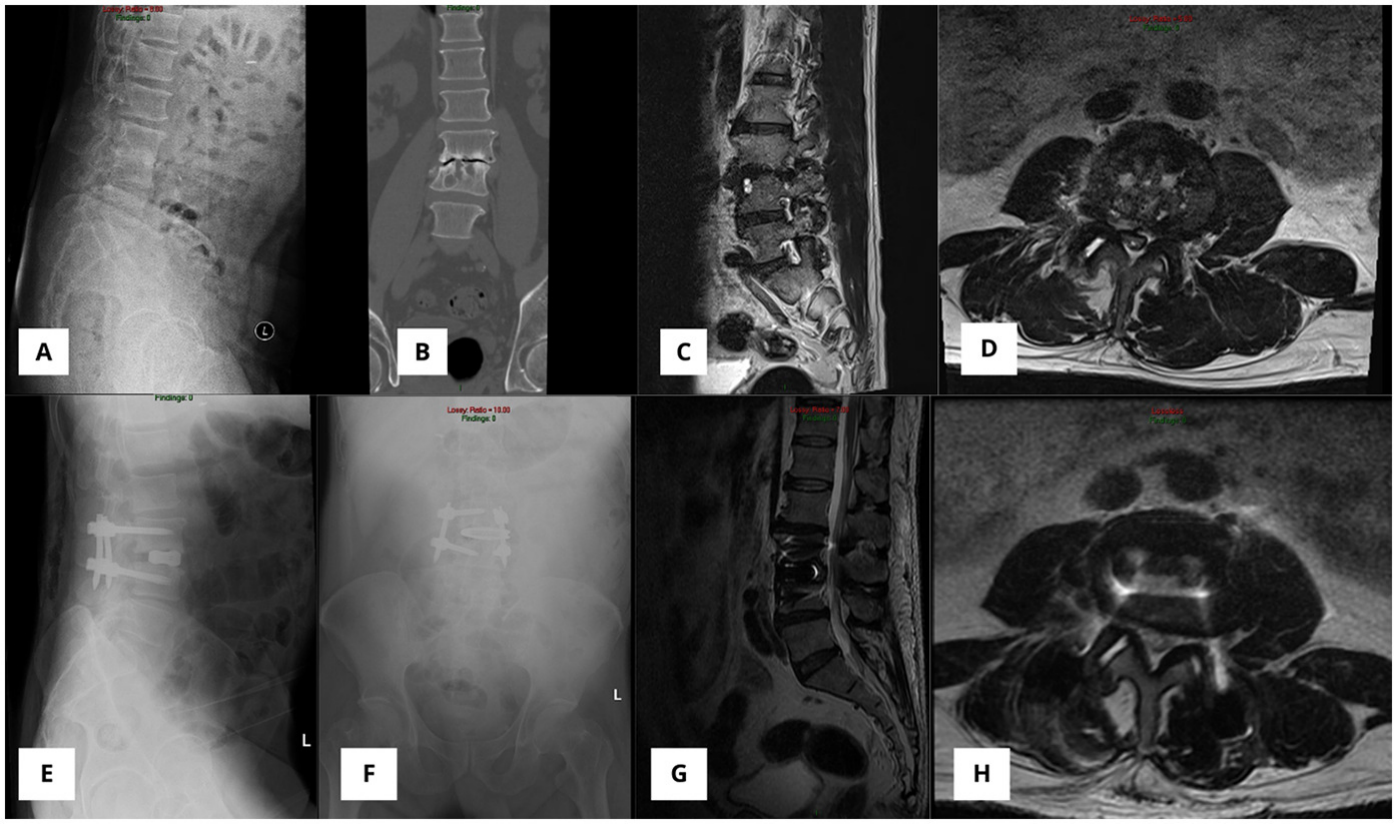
(A) X-ray image in lateral extension showing disc narrowing in L3/4 before surgery. (B) CT scan showing disc narrowing in L3/L4. (C,D) MRI showed disc narrowing at the level of L3/4. Postoperative x-ray (E,F) and MRI (G,H) showed that the intervertebral cage was well positioned without displacement.
